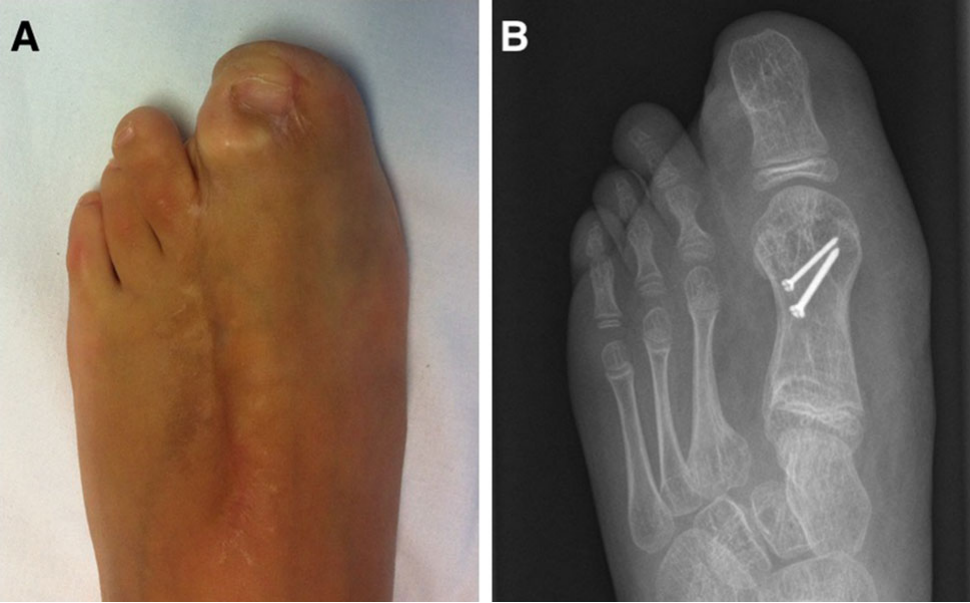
Case 1: Clinical photograph (a) and radiograph (b) at 15 months follow-up
